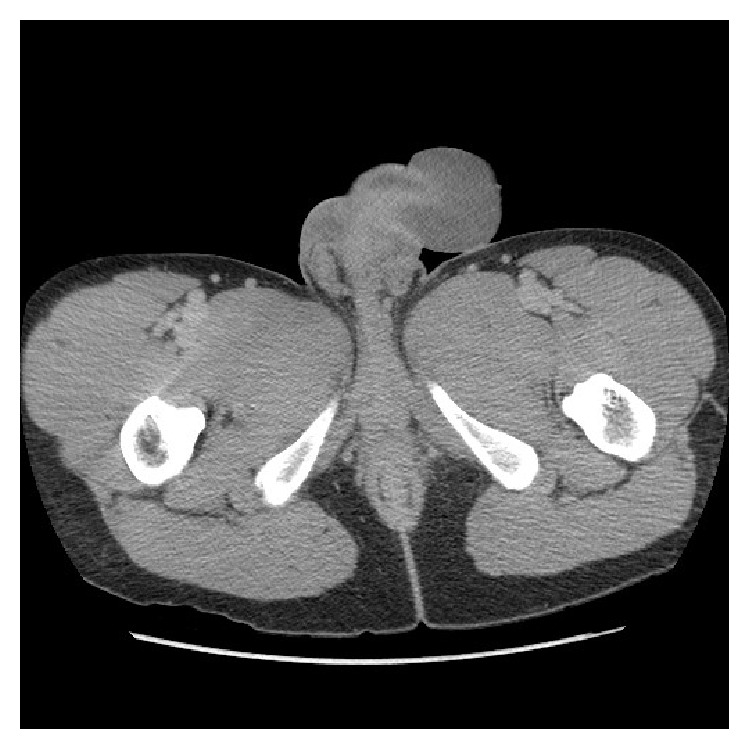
Contrast CT scan of the pelvis of Case 2. CT scan showed diffuse edematous thickening of the skin of the penis without evidence of focal fluid collection or abscess.
